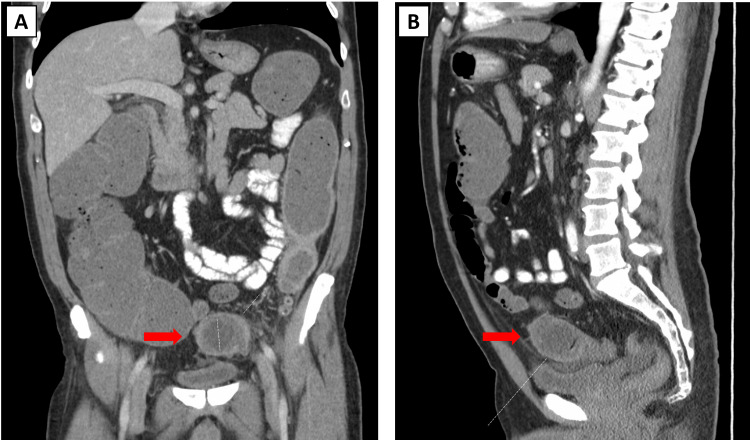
CT scan of the abdomen showing the gallstone: a round, non-calcified hypodense structure with eccentric air impacted in the proximal sigmoid colon. Red arrows indicate the gallstone: (A) coronal plane; (B) sagittal plane. CT, computed tomography
